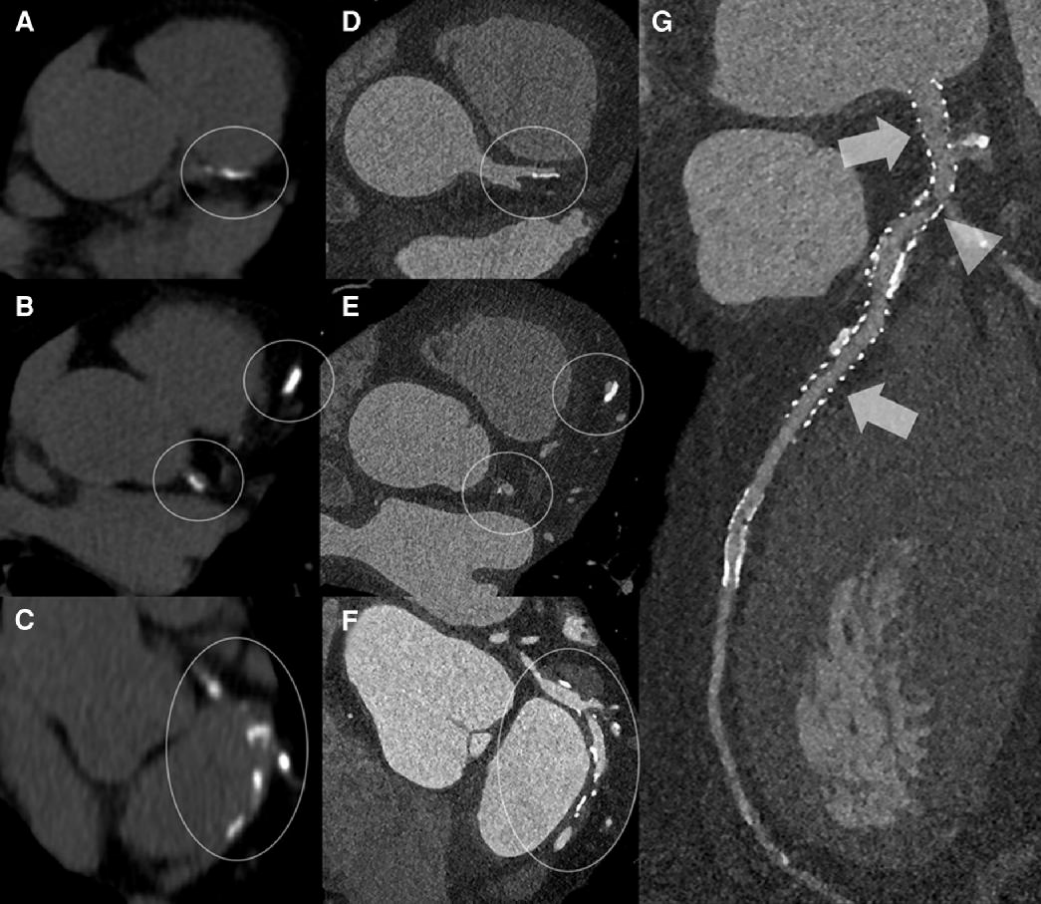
The figure shows examples of CAD as displayed by conventional calcium scoring protocol (*A*, *B*, *C*; inside white circles and ellipses) and corresponding CT coronary angiography images (*D*, *E*, *F*; inside white circles and ellipses) obtained with photon counting CT—PCCT technology (NAEOTOM ALPHA, Siemens Healthineers). It is very clear that the detection and phenotyping of calcifications is going to be dramatically improved by this technology. These improvements will also determine a much better visualization of high attenuation/density structures such as coronary artery stents (*G*) in which even stent structs will be easily differentiated (arrows) together with in-stent re-stenosis (arrow-head).
